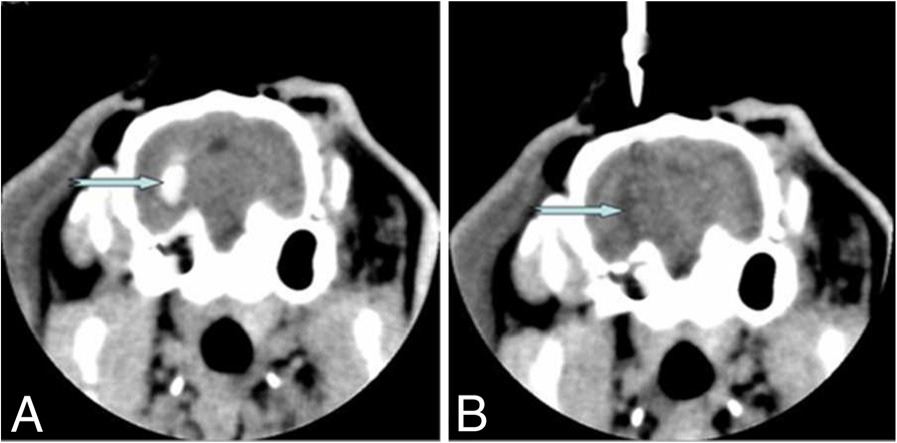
Brain CT showing changes of intracranial hematoma before and after the minimally invasive procedures. A: Basal ganglia hematoma was observed after the ICH model was prepared successfully. B: The intracerebral hematoma disappeared after the MI procedures were performed.
